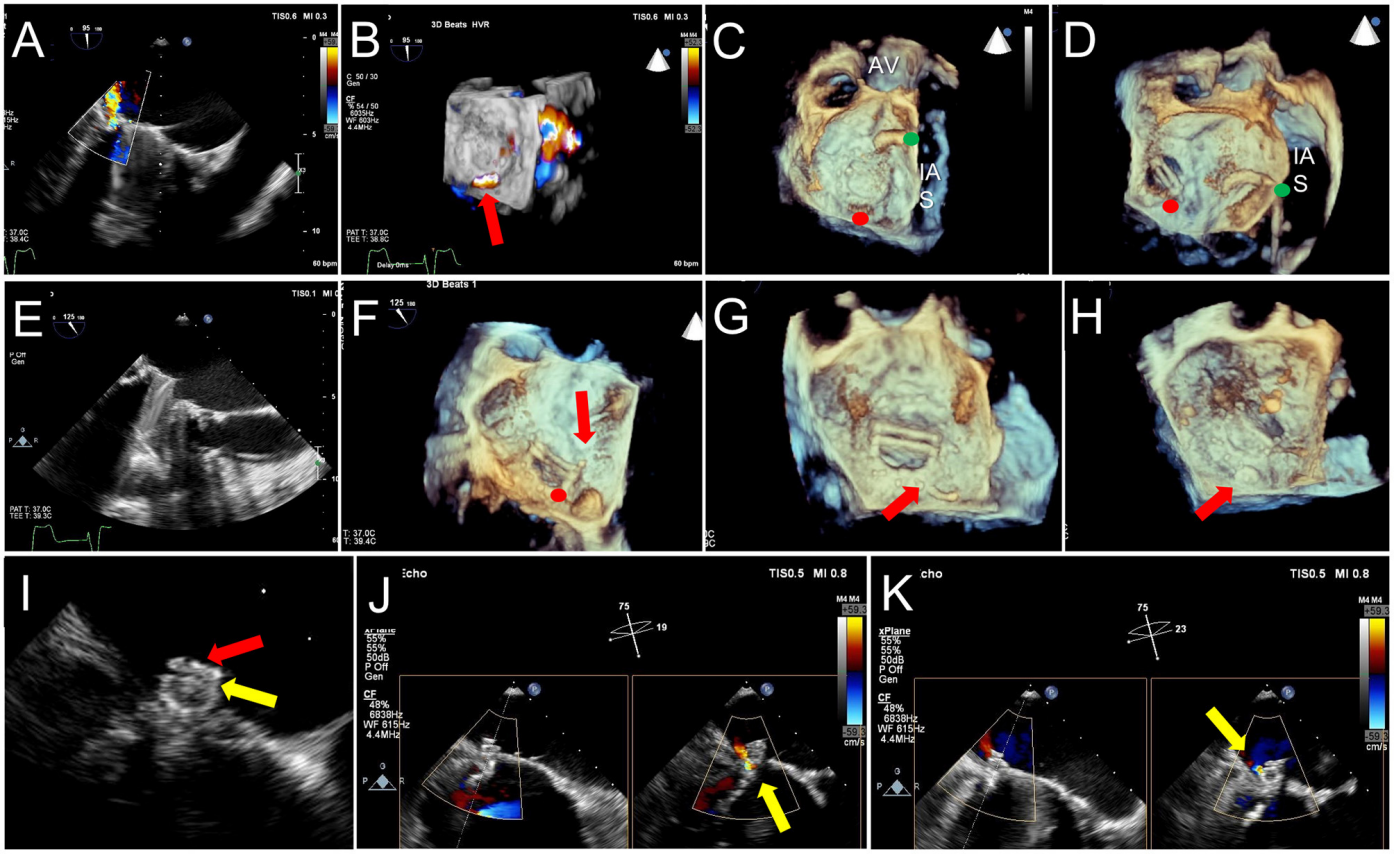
TEE Guidance of Challenging Closure of Posteromedial PVL. A posterior paravalvular leak resulting in hemolysis is visualized by 2D color Doppler (A) and 3D color Doppler Imaging (between 5 and 6 o clock in a surgeon's view, red arrow, B). (C) The first attempt at transseptal puncture is shown (green circle). The location of PVL is marked by a red circle. (D) After unsuccessful attempts to cross the defect, the septum was repunctured more posterior (green circle) for a better trajectory toward the defect. (E) After additional unsuccessful attempts, the approach was changed to retrograde. (F) As acoustic shadowing limits visualization on the ventricular aspect of the mechanical mitral prosthesis, wire exit point was visualized in the left atrium. Here, the wire is visualized inside the sewing ring (red arrow) adjacent to the PVL (red circle). (G) After reorientation of the wire, the defect was successfully crossed (red arrow). (H) The atrial disk of an AVPII closure device is visualized after deployment on 3D imaging (red arrow). (I) 2D echocardiography demonstrates the atrial disk (red arrow) and a portion of the center cylinder (yellow arrow). (J) A residual peri-device jet is seen (yellow arrow). (K) After device re-sheathing and slight repositioning, there is a trivial residual PVL jet flowing through the center of the closure device (yellow arrow). AV, aortic valve; IAS, interatrial septum.
